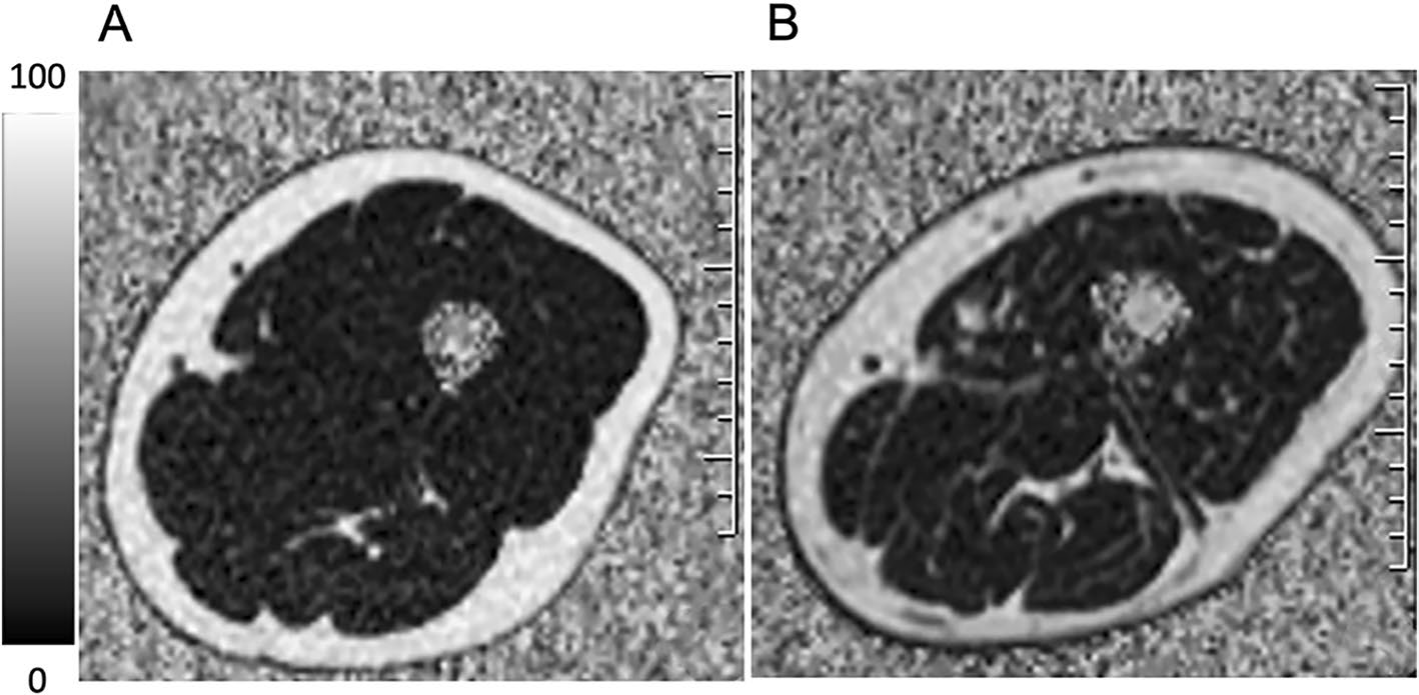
Representative MRI PDFF of thigh in control and T2DM. (A) Control volunteer without T2DM, Female, 55 years old. IMAT PDFF_extensors_ = 10.60%, IMF PDFF_extensors_ = 2.75%, IMAT PDFF_flexors_ = 17.00%, IMF PDFF_flexors_ = 4.10%. (B) T2DM patient, Female, 55 years old. IMAT PDFF_extensors_ = 18.90%, IMF PDFF_extensors_ = 6.05%, IMAT PDFF_flexors_ = 23.08%, IMF PDFF_flexors_ = 10.57%. White represents high PDFF values.
